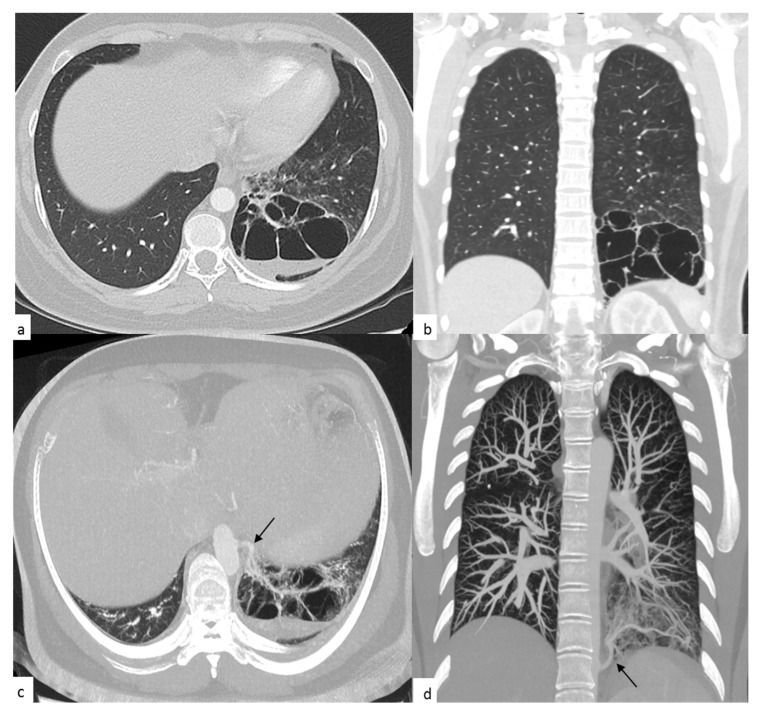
Axial (a,c) and coronal (b,d) chest computed axial tomography (CT) scan: (a) and (b): images with contrast show a hypodense, cystic avascular lesion, with multiple internal septa (multicystic) and diameters greater than 67 × 69 × 88 mm (PA × T × L); the cysts measure between 15 and 35 mm, are located in the posterior segment of the left lower lobe, and contain air-fluid levels. Peripheral parenchyma bronchial wall thickening and centrilobular micronodules are also evidenced. (c) and (d): images obtained using multiplanar reformation (MPR) outline the course of an aberrant arterial vessel (black arrow), which originates from the left lateral aspect of the thoracic aorta and supplies the left basal cystic lesion.
